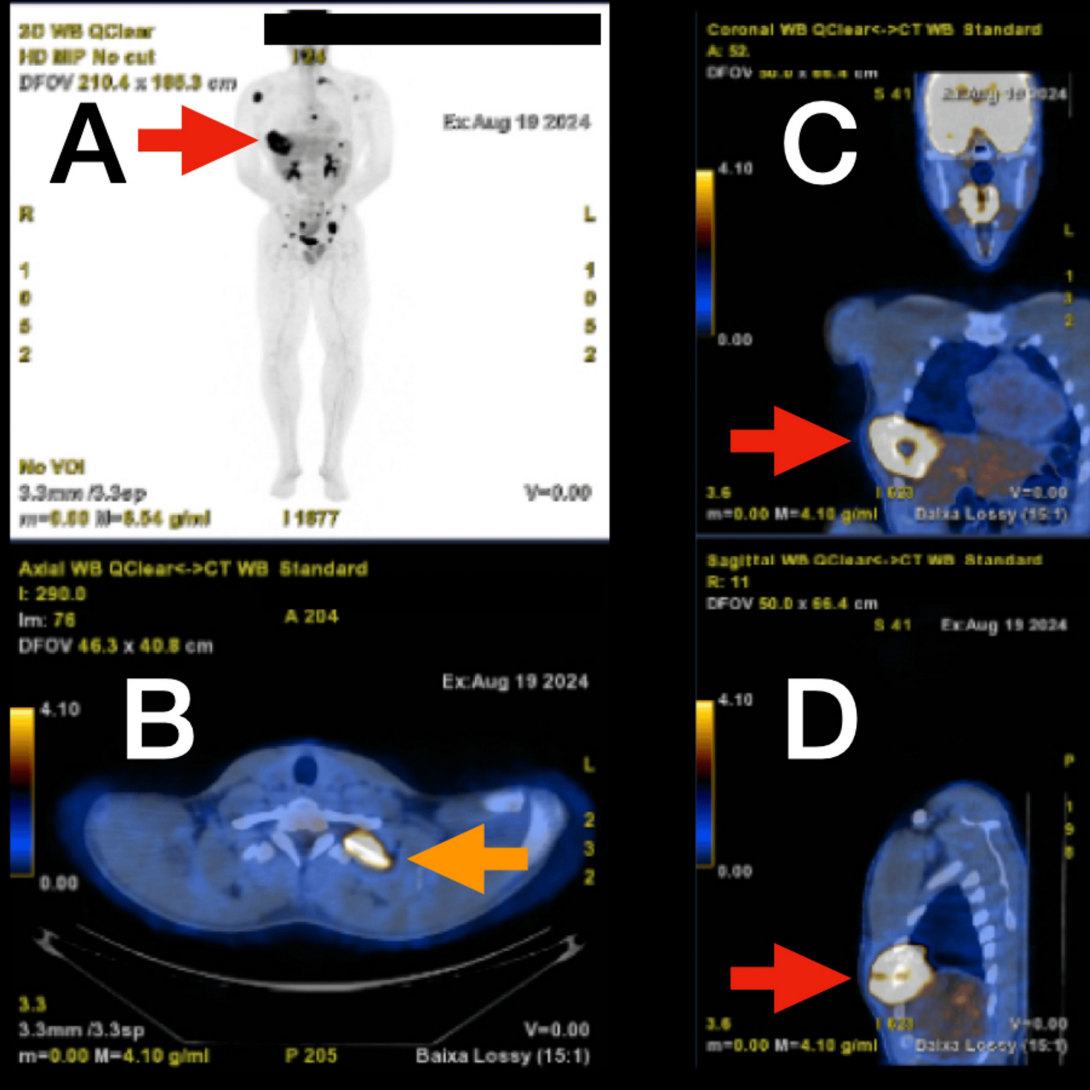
Whole-body PET scan with FDG Panel A shows the primary lesion surrounded by widespread skeletal metastases (red arrow). Panel B shows intense FDG uptake in the secondary lesion on the left second rib (orange arrow). Panels C and D show intense FDG uptake in the primary rib lesion (red arrow). PET: positron emission tomography; FDG: fluorodeoxyglucose
